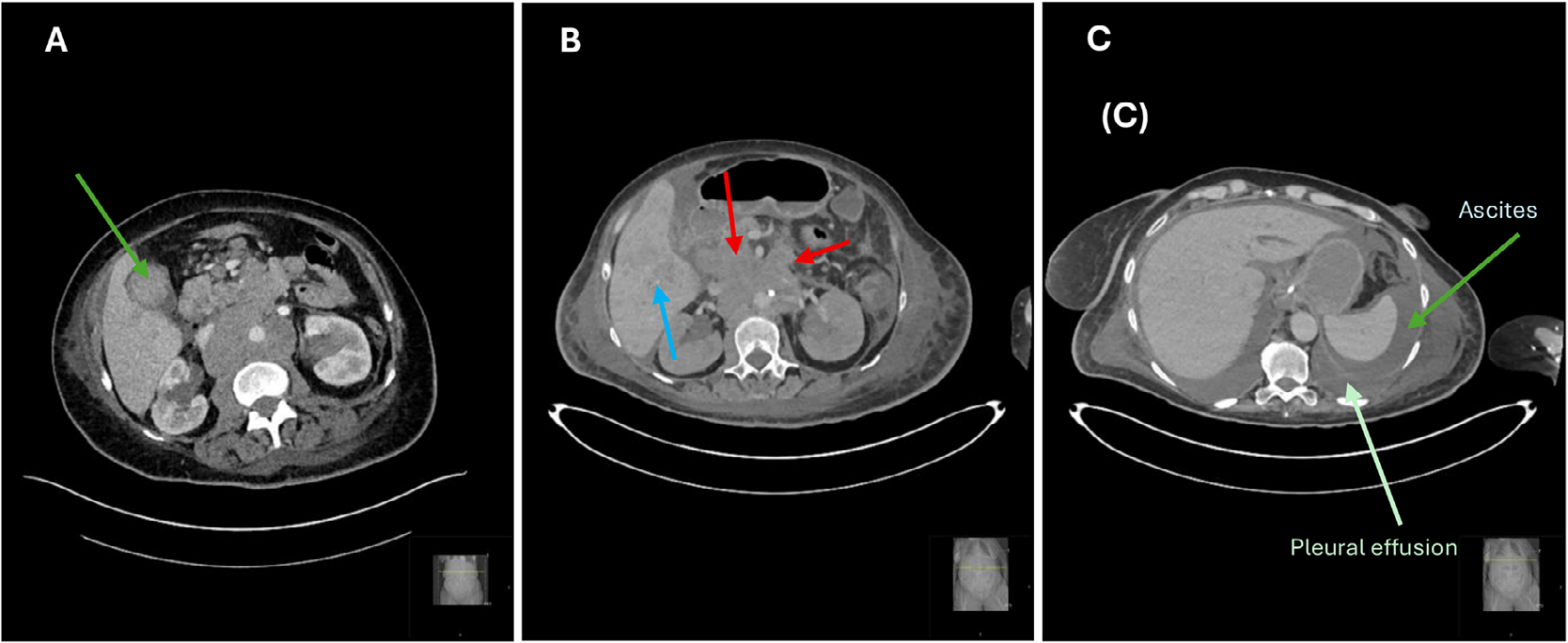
(A) Follow‐up CT scan showing a solid mass replacing the gallbladder (green arrow). (B) Axial CT showing multiple new subcentimeter low‐attenuation lesions throughout the right hepatic lobe (blue arrow). Findings are concerning for metastatic liver involvement. Progressive retroperitoneal lymphadenopathy (red arrows). (C) Axial CT image showing ascites and bilateral pleural effusions.
